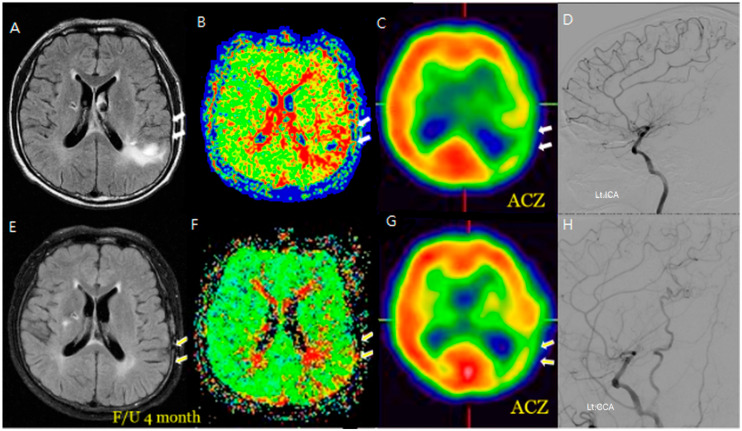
An M/58 symptomatic steno-occlusive patient (Rt. hemiparesis with Lt. M1 occlusion). Preoperative MR FLAIR, perfusion MR, and SPECT (A–D) show hyperintense vessels, indicating a decreased cerebral vascular reserve; a postoperative decrease or absence of hyperintense vessels reveals successful revascularization for decreasing HV after F/U; and a decrease in HV on the bypass-established hemisphere is associated with an improved hemodynamic status (E–H). Hyperintense vessels in preoperative FLAIR (A) coincide with a perfusion delay on SPECT (C). Postoperatively (E–H), the HV resolution matches with improved perfusion, demonstrating spatial and functional improvement.
